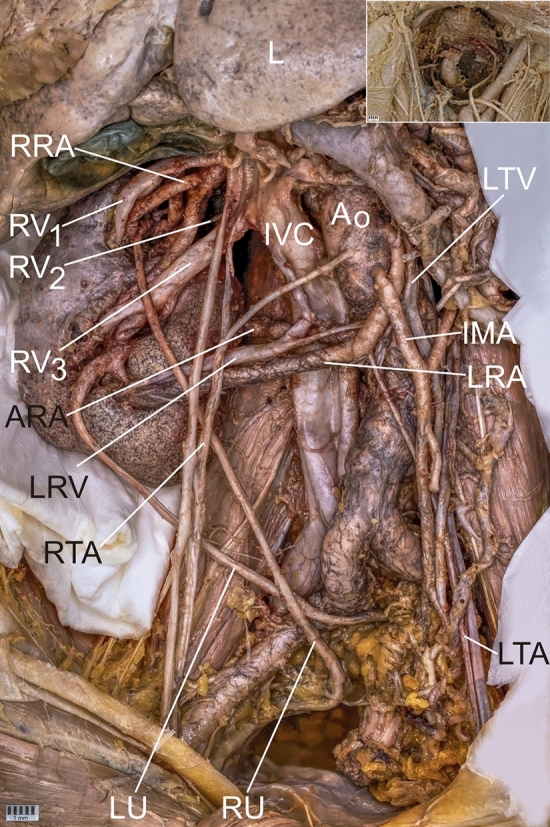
Abdominal vessels and ectopic kidney with the additional perspective of the pelvic cavity in the corner. *AG* Adrenal gland, *Ao* Aorta, *ARA* Accessory renal artery, *CFRE* Crossed fused renal ectopia, *CT* Celiac Trunk, *IMA* Inferior Mesenteric Artery, *IVC* Inferior Vena Cava, *L* Liver, *LRA* Left Renal Artery, *LTA* Left Testicular Artery, *LRV* Left renal vein, *LU* Left ureter, *RRA* Right renal artery, *RTA* Right Testicular Artery, *RU* Right ureter, *RV* Renal Vein, *SMA* Superior mesenteric artery
